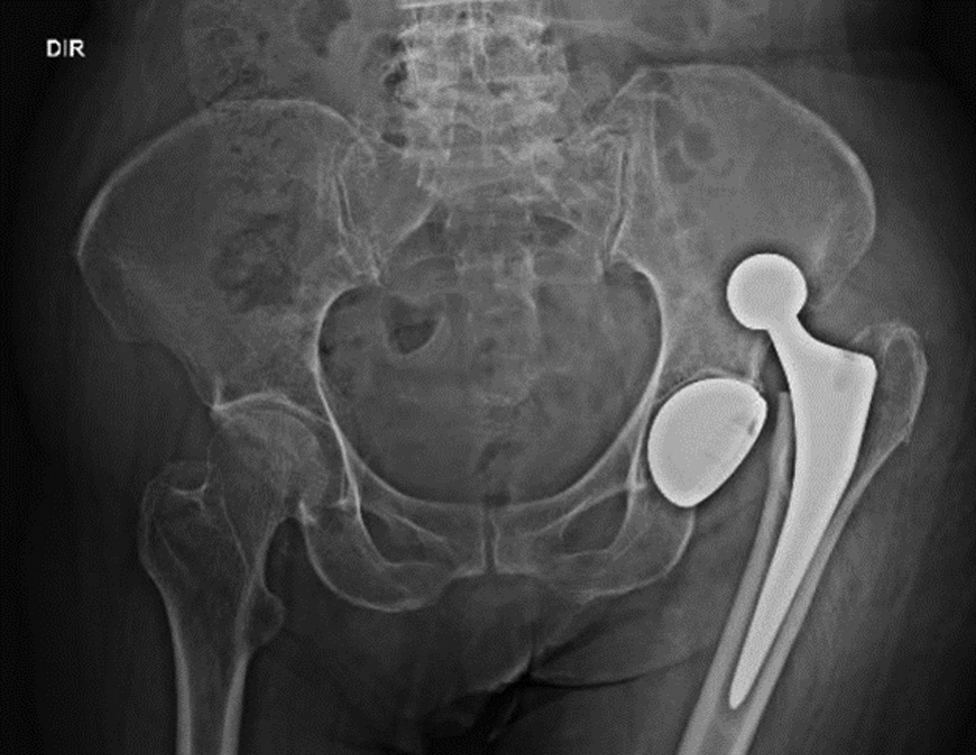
Panoramic radiography of the basin showing posterosuperior dislocation of the left hip prosthesis.
